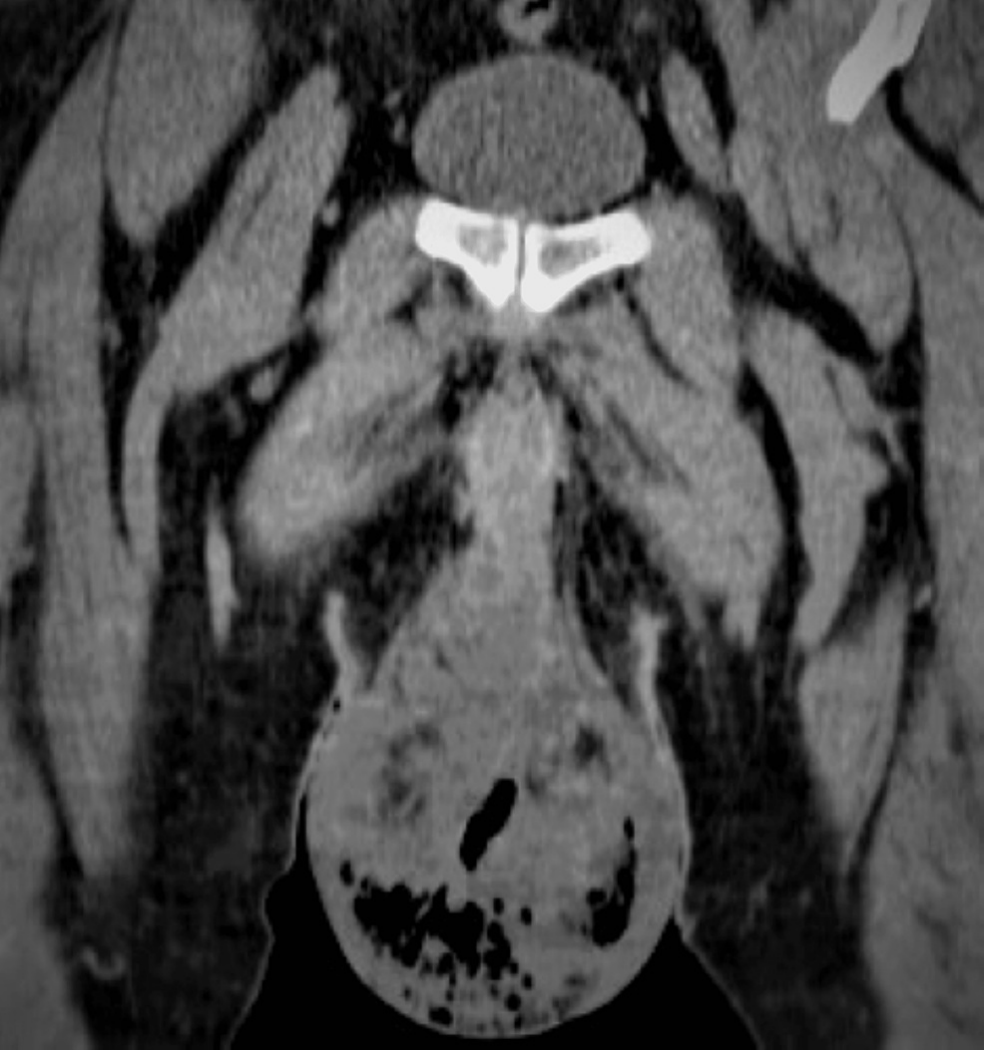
Coronal CT image of the pelvis showing gas- and fluid-containing bilateral scrotal infection.
